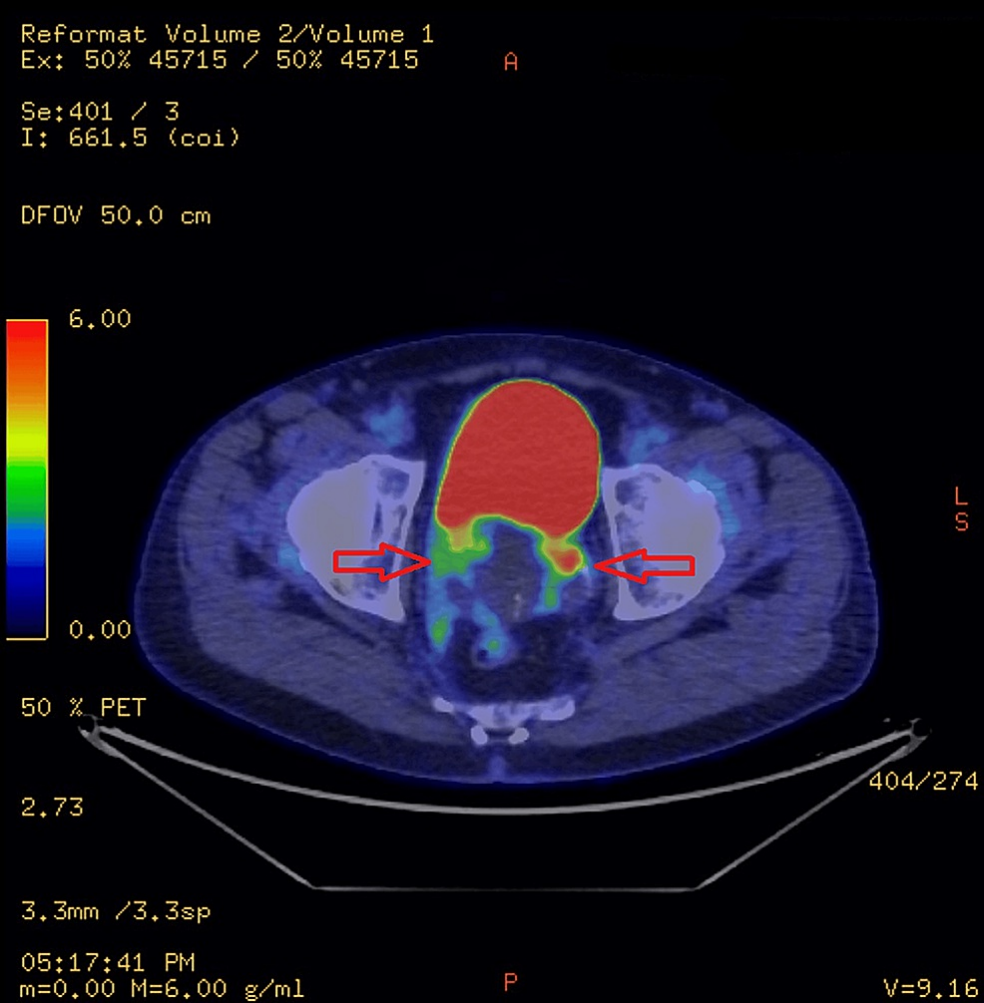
FDG uptake within the left lobe of the prostate and seminal vesicles
